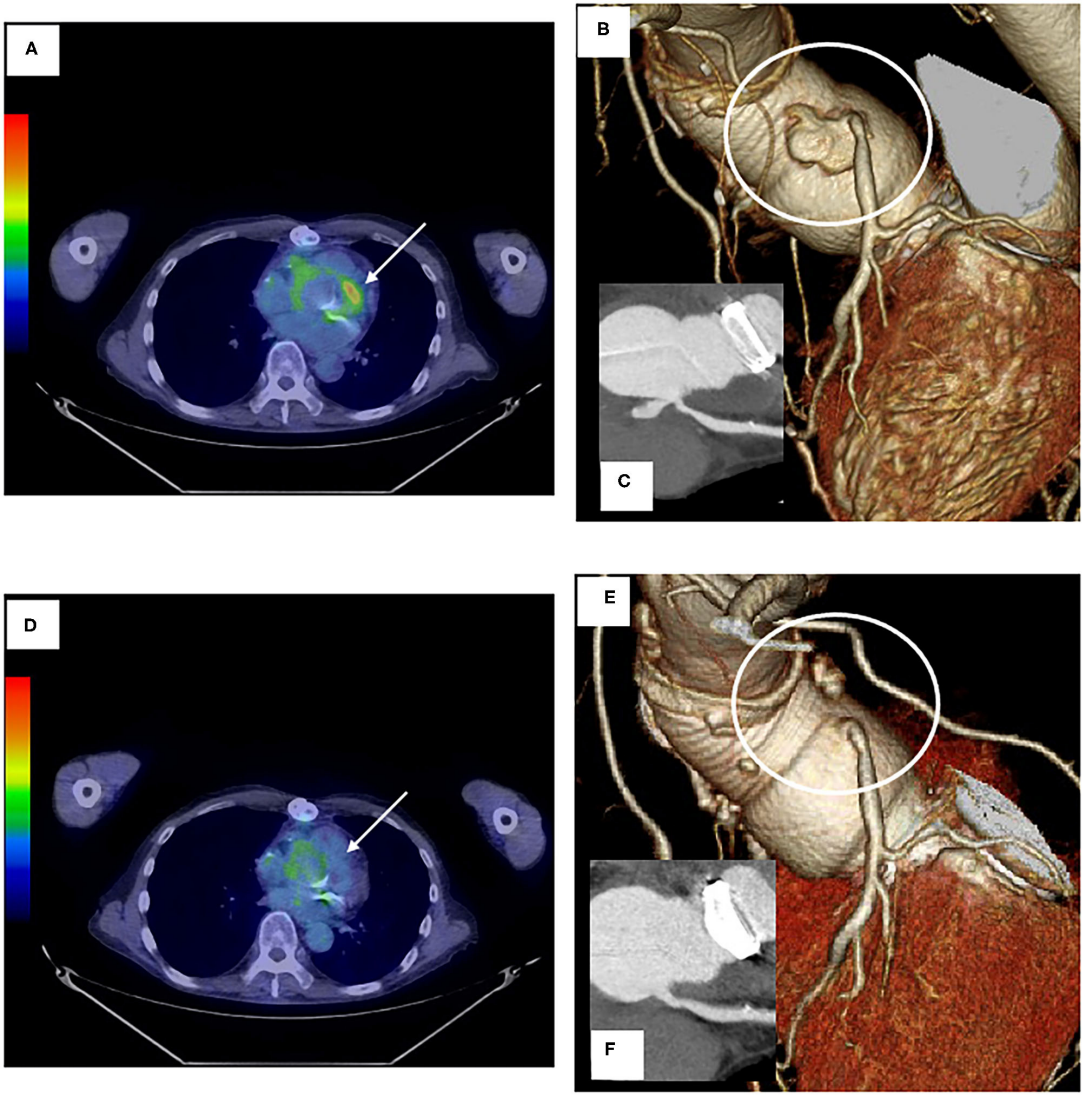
(A,D) Images of ^18^F-fluorodeoxyglucose (^18^F-FDG) positron emission tomography/computed tomography before (A) and after (D) steroid therapy. Arrows indicate the anastomotic site with left coronary artery aneurysm. (B,C,E,F) 3D coronary angiography and contrast-enhanced CT images before (B,C) and after steroid administration (E,F).
